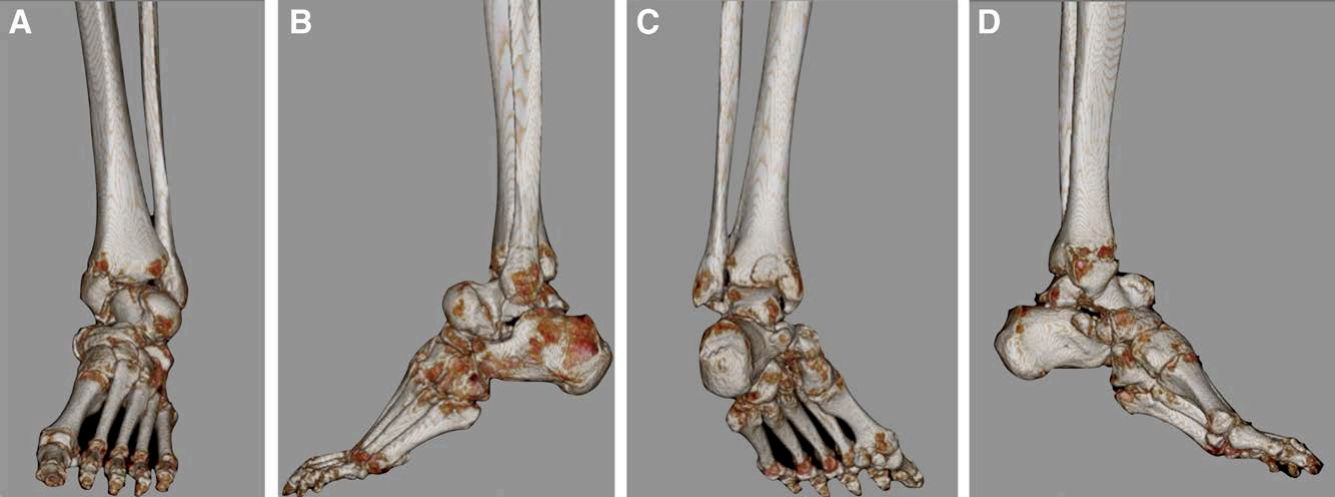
Musculoskeletal computed tomography (CT) scan with three-dimensional reconstruction of the left foot and ankle showing pan-talar dislocation (subtalar, talonavicular, and tibiotalar joint) and body fracture; and displaced medial malleolar fragment with disrupted medial side mortise, the posterior malleolar fragment involved <25% of the articular surface with <2 mm displacement, and the lateral malleolar Danis–Weber type B fracture with a congruent lateral side mortise.
